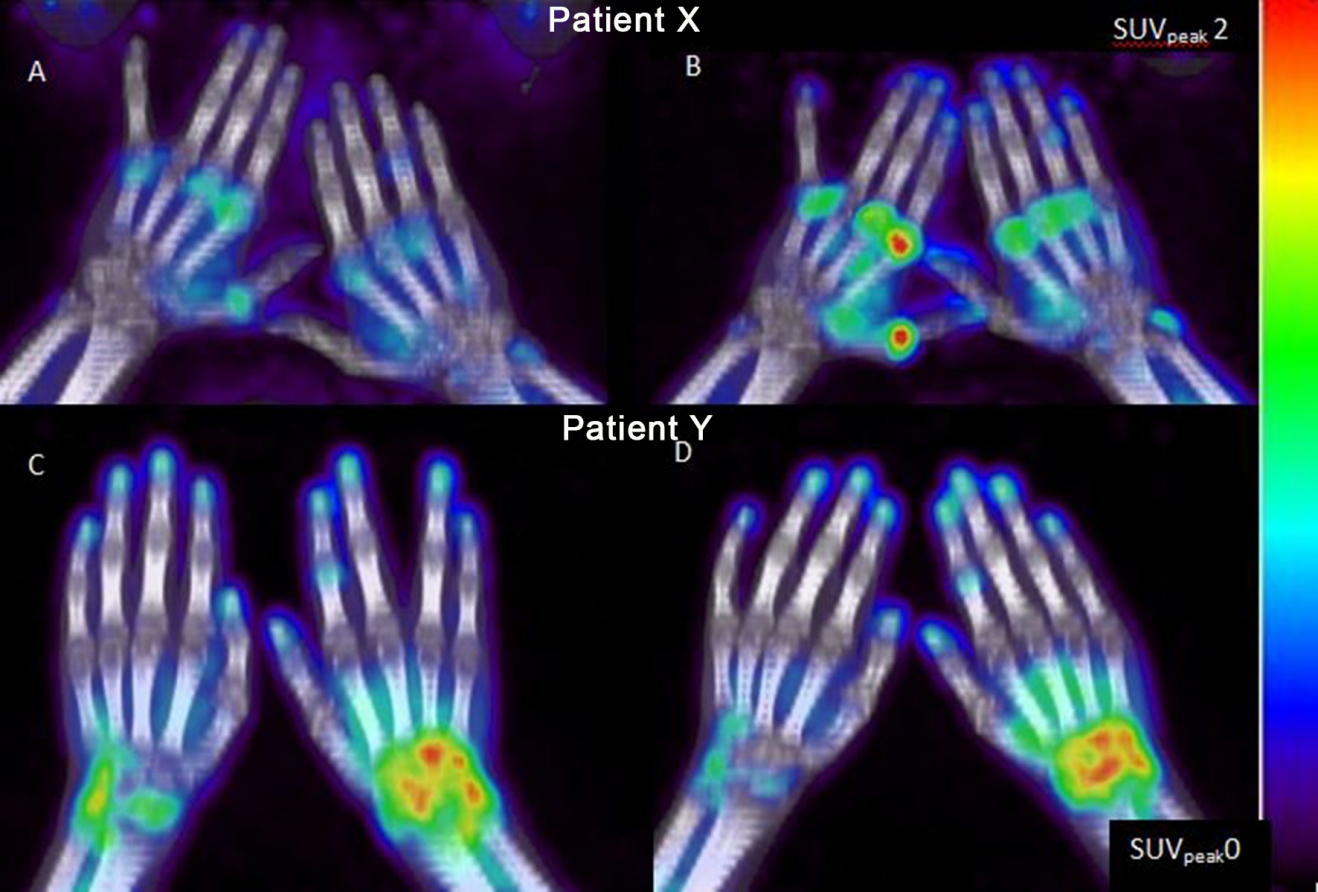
PET/CT Maximum Intensity Pictures show accumulation of the three TSPO tracers. (A) *(R)*-[^11^C]PK11195 and (B) [^18^F]DPA-714 images within one patient (patient X), and (C) [^18^F]DPA-714 and (D) [^11^C]DPA-713 images within another patient (patient Y).
